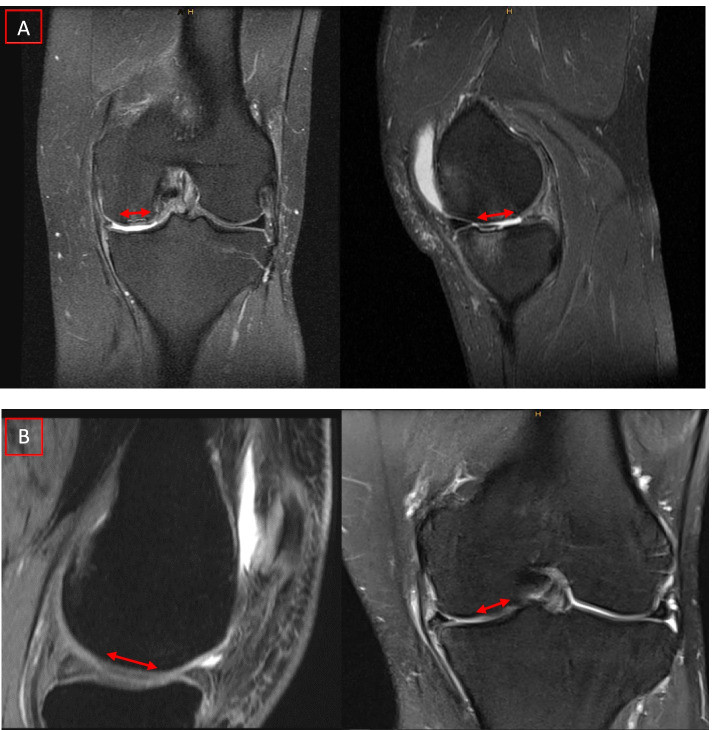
Examples of cartilage defect assessment on MRI and grading according to the AMADEUS instruction guide. A) Example of a full thickness cartilage defect with an associated bone marrow edema and small (< 5 mm bony defect) scoring for an AMADEUS grade IV. B) Example of a superficial cartilage defect with intact subchondral bone and absent bone marrow edema scoring for an AMADEUS grade II
